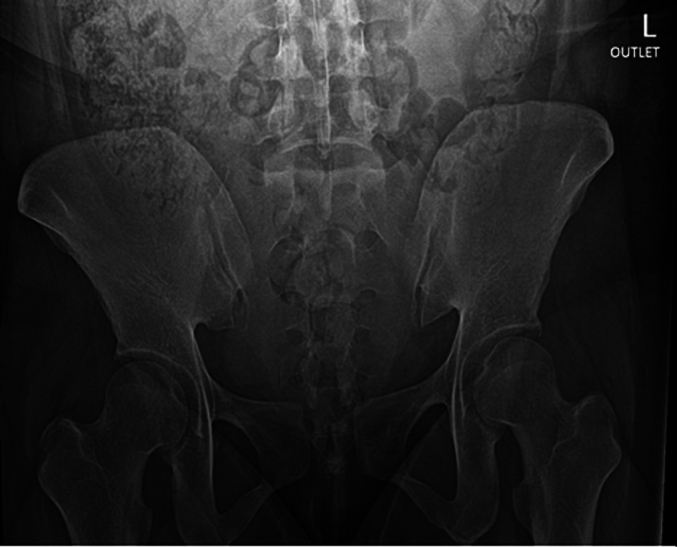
x-ray; an AP outlet view of the pelvic.
